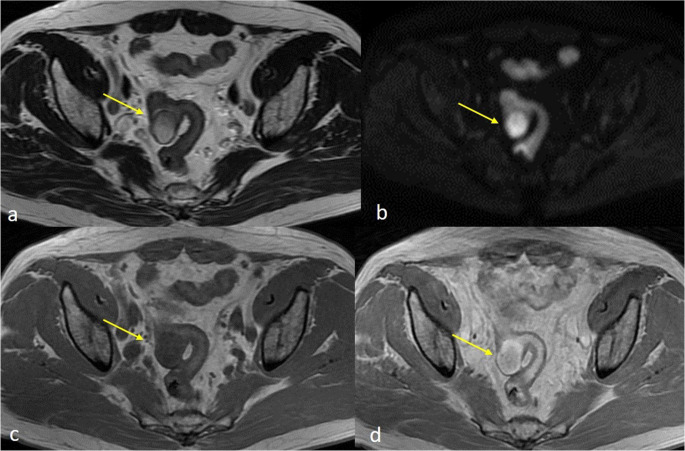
Abdominal MRI. (A) T2-weighted images show a hyperintense intramural sigmoid mass (yellow arrow) (B) with high B value in DW images (yellow arrow). (C) T1-weighted images before iv contrast administration show a hypointense intramural mass (yellow arrow) (D) T1WI post-contrast study, show heterogeneous enhancement of the mass. No lymphadenopathy was noticed (yellow arrow)
